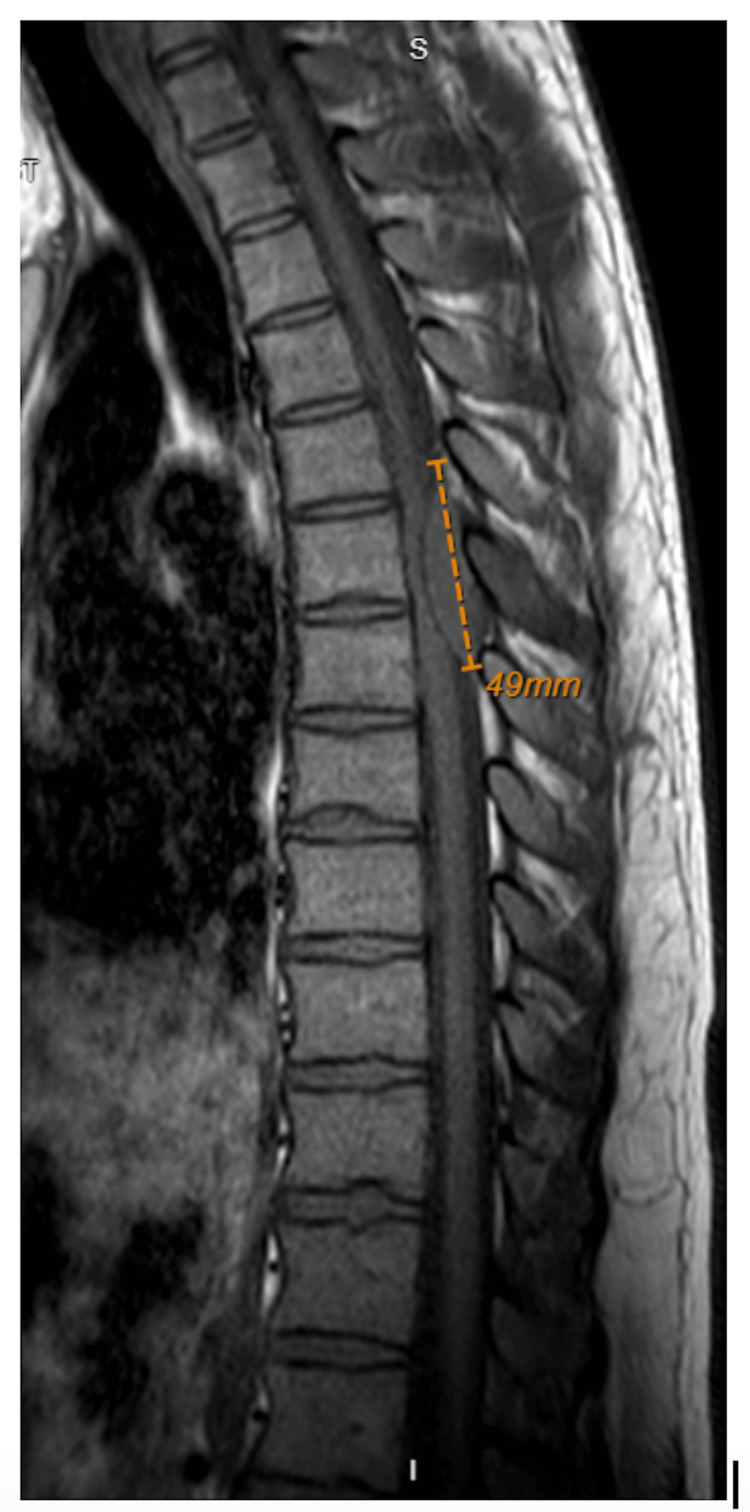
Thoracic MRI with sagittal view showing a 4.9 cm posterior epidural mass at T6-T7
